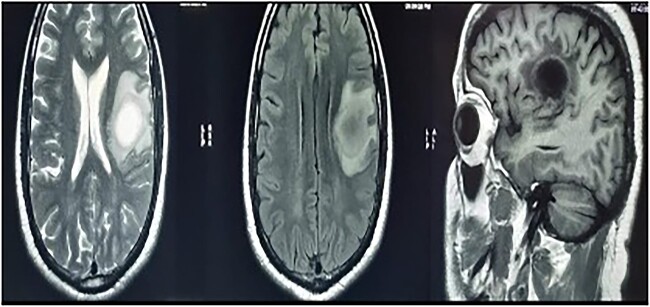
MRI brain with contrast—a heterogeneous irregular rim of an enhancing lesion with a size of 3.6 × 3.4 × 2.4 cm appearing in the left frontal subcortical region, with mild perilesional edema extending into the corona radiata and external capsule.
